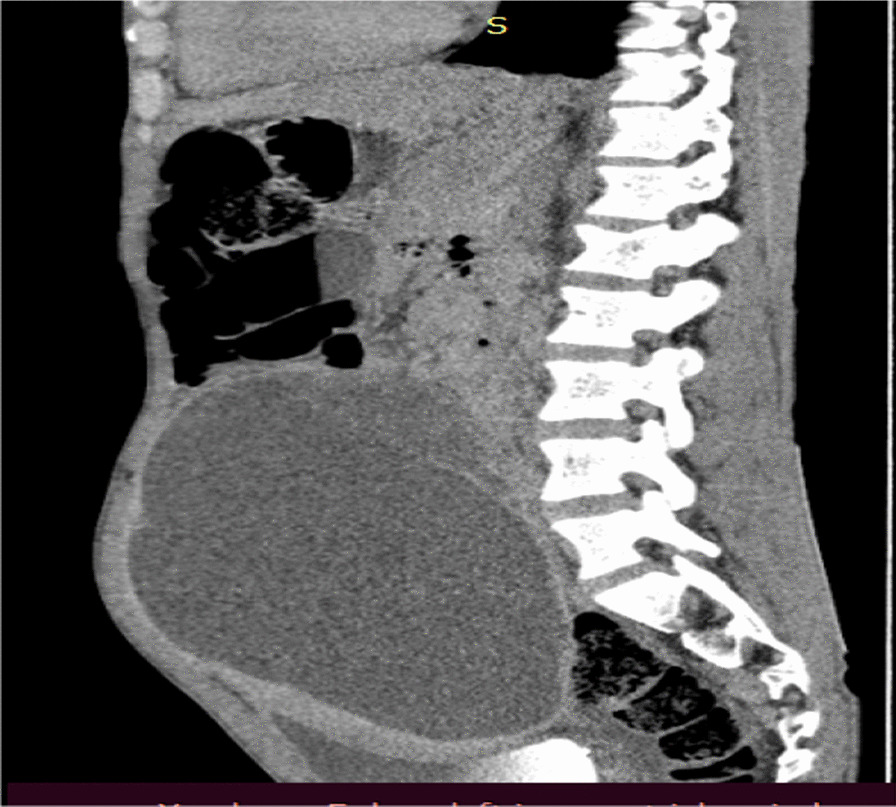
Sagittal view of the abdominal CT scan of the patient
